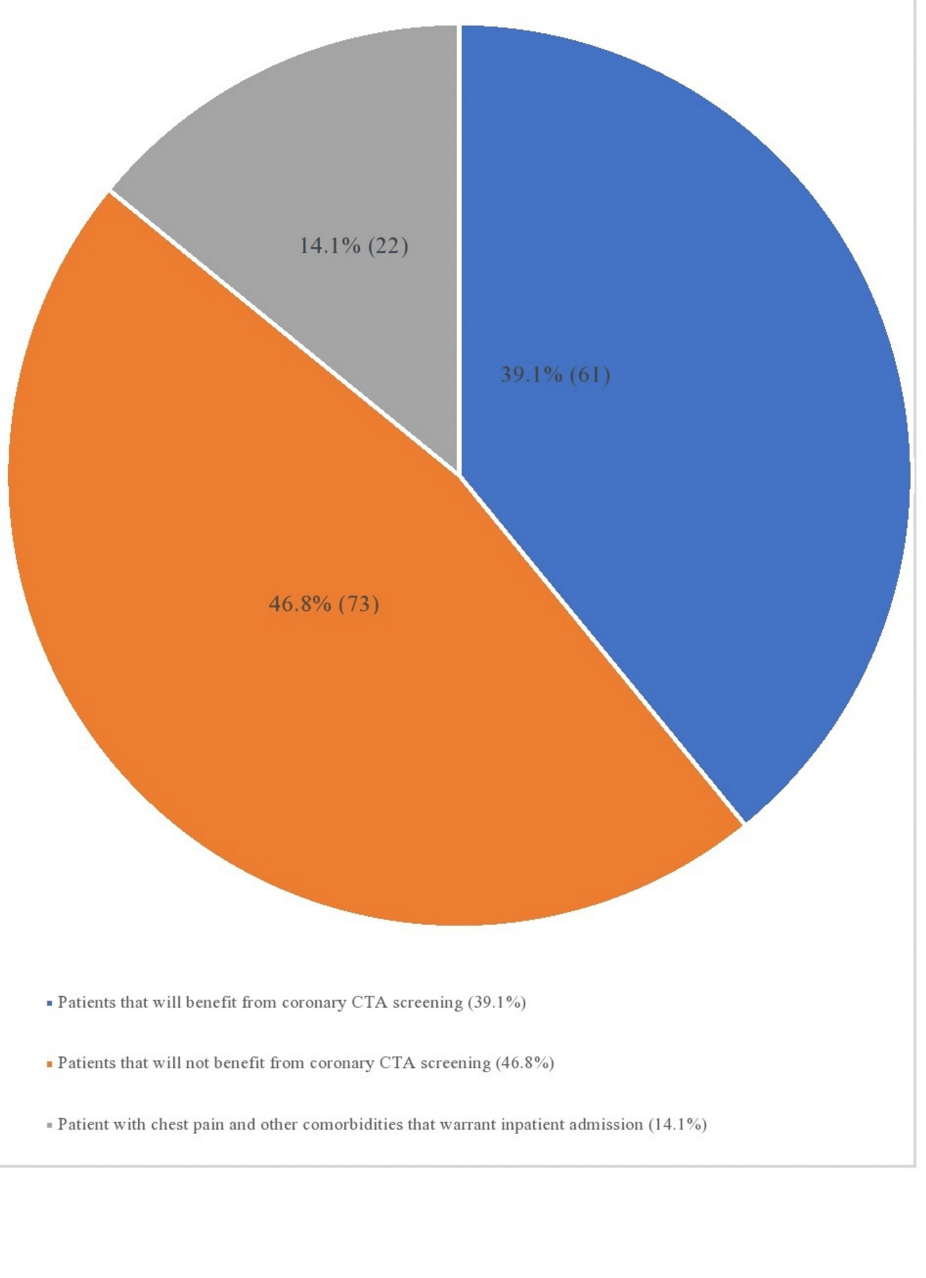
Further breakdown showing patients who would have benefited from coronary CTA screening CTA: Computed tomography angiography
